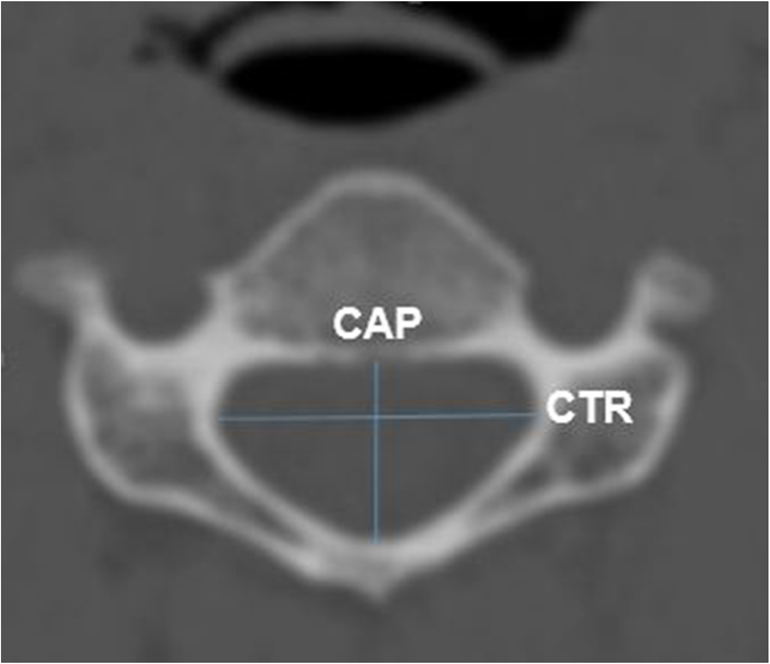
Measurements of CAP and CTR on axial CT image
